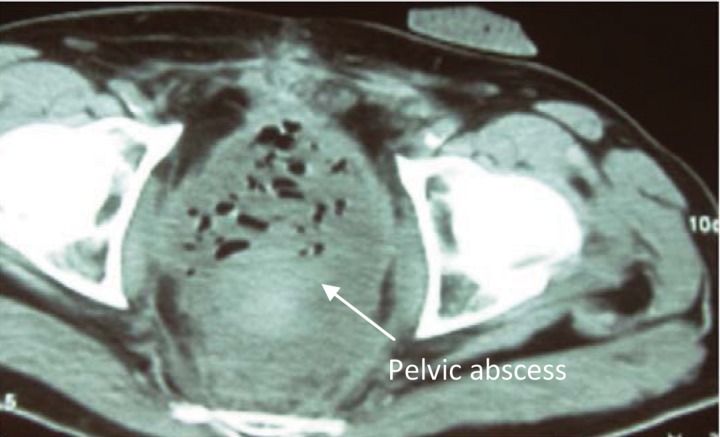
Axial computed tomography of the pelvis showing pelvic abscess
